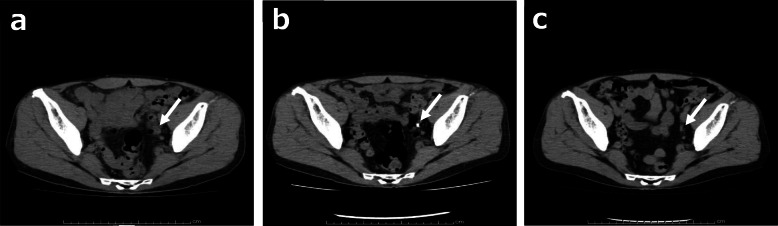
CT findings for the ureteral lesion. The white arrows indicate the left ureteral lesion. a. Before administration of PSL. b. Eighteen days after the initiation of PSL, complete resolution of the left ureteral lesion was recognized. c. Sixty-three days after the initiation of PSL, there was no recurrence after the removal of DJS. CT: computed tomography, PSL: prednisolone, DJS: double J stent
